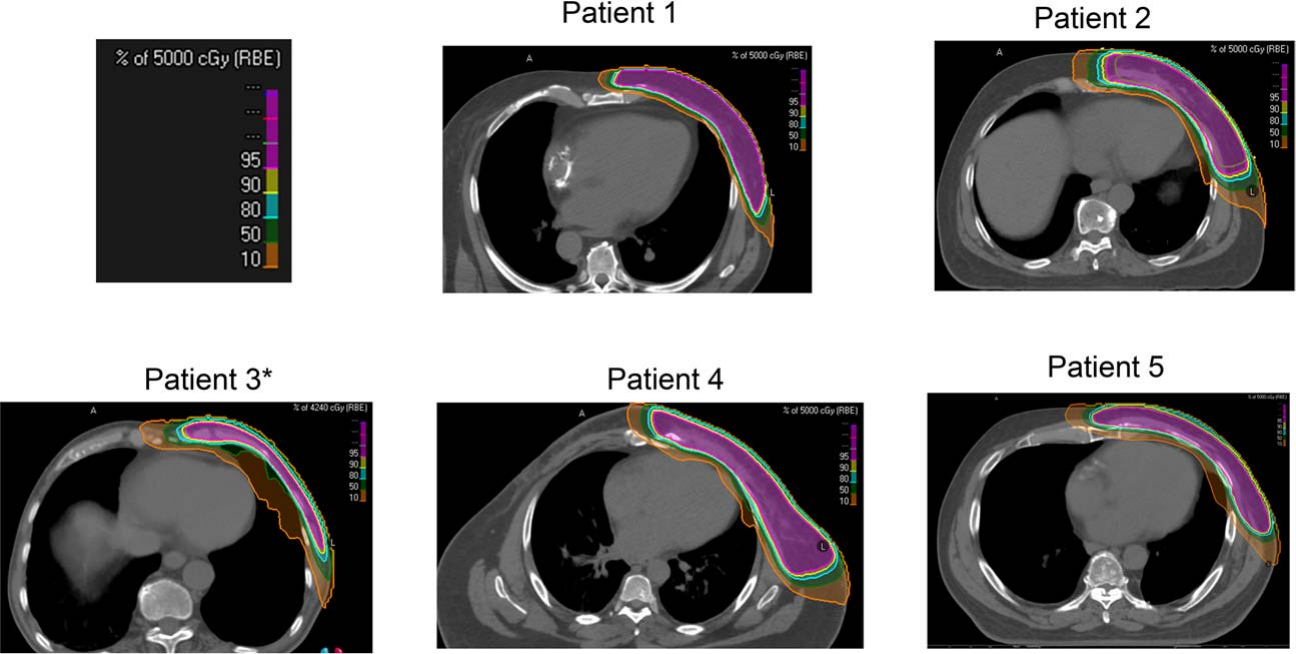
The radiation proton dosimetry for each patient on a representative axial CT slice, shown in colorwash by percentage isodose line for the initial phase (prescription dose of 50 Gy for patients 1, 2, 4, and 5; and 42.4 Gy for patient 3): 95%, pink; 90%, yellow; 80%, light blue; 50%, green; 10%, orange. *This patient’s plan shows the percentage isodose lines for a prescription dose of 42.4 Gy. Abbrevations: CT, computed tomography; Gy, Gray.
